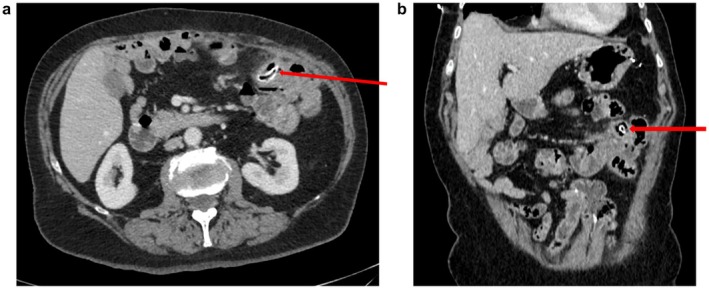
(a) Axial contrast‐enhanced CT image from the initial scan demonstrates a well‐defined, tubular hyperdense foreign body (red arrow) located within a loop of the jejunum in the right mid‐abdomen. The foreign body appears oriented transversely, partially surrounded by intraluminal fluid and gas. There is no definitive evidence of free intraperitoneal air or localized fluid collection to suggest perforation at this stage. The surrounding mesenteric fat shows no stranding, and there is no associated bowel wall thickening or mucosal disruption evident on this initial scan. These findings suggest early or non‐complicated impaction of the foreign body without acute inflammatory changes. (b) Sagittal reconstruction from the initial contrast‐enhanced CT scan, clearly delineating the intraluminal position of the tubular foreign body (red arrow) within a distended jejunal loop. The foreign object is oriented longitudinally in this plane and appears to maintain a fixed position relative to surrounding bowel loops. There is no discernible evidence of transmural extension or perforation. The adjacent mesenteric fat appears preserved without stranding, and the serosal surface of the bowel loop is smooth, further supporting a non‐complicated foreign body impaction at the time of imaging.
